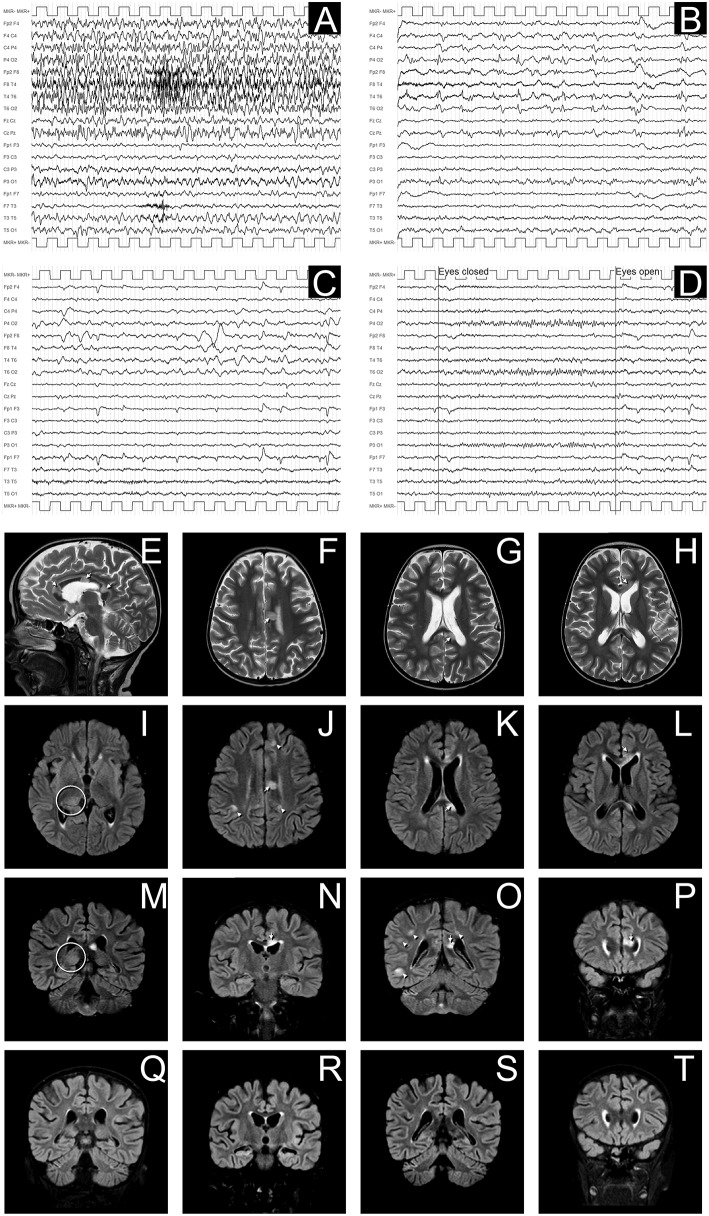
EEG and MRI evolution of encephalitic episode. (A–D) EEG recordings in longitudinal bipolar montage, 15 s each panel, calibration (MKR) amplitude 100 μV. In (A) a clear-cut ongoing epileptic discharge involving the right hemisphere (mainly the infrasylvian derivations) is documented, clinically associated with the sub-acute onset of neurological symptoms, consistent with the diagnosis of focal Non-Convulsive Status Epilepticus (NCSE). In (B) the recording obtained 8 h later, after intravenous levetiracetam and phenytoin treatment, shows the resolution of the NCSE with residual diffuse slowing (predominant on the right hemisphere) and periodic temporo-occipital sharp transients (more evident on P4-O2, T4-T6, and T6-O2 channels). Ten days after sub-acute onset, the EEG does not show epileptiform discharges, but an increased amount of slow activity remains, with obvious preponderance on the right temporal regions (C). In (D) the EEG recording performed after 3 months demonstrates a complete recovery, with normal reactivity of the posterior background alpha rhythm. (E–P): Brain MRI images obtained at the onset of neurological symptoms, demonstrating multiple focal hyperintense lesions in T2-weighted (sagittal plane in E; axial images in F–H) and FLAIR (axial images I–L; coronal plane in M–P) sequences. The largest 3 lesions were located in corpus callosum (arrows in E–H,J–L,N–P). A clear hyperintensity was also visible on the right posterior thalamus (circles in I,M). Multiple lesions were evident at the cortico-subcortical junction bilaterally (arrowheads in J,O), mainly involving the right hemisphere, being the largest in the right temporo-occipital region (O). The MRI performed 30 days after the encephalitic episode showed a complete resolution of the focal lesions (in panels Q–T are depicted the correspondent coronal FLAIR images as in M–P).
